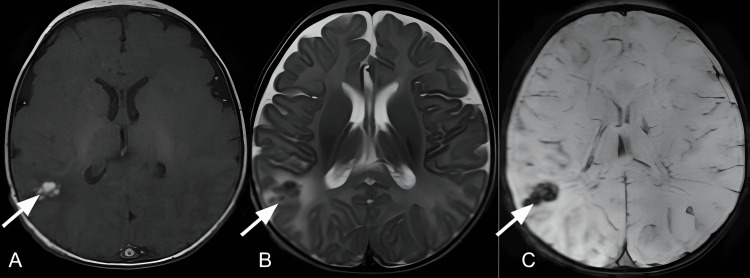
Brain MRI Axial T1- (A), T2-weighted images (B) and susceptibility weighted imaging (C) showed a right parietal subcortical hematoma with mild surrounding vasogenic edema.
